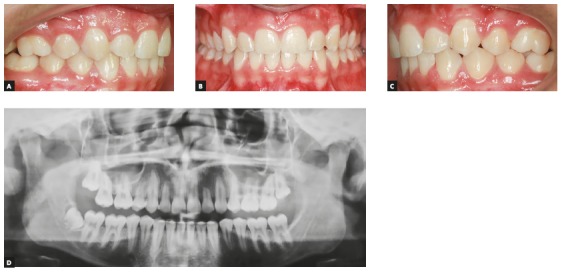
A, B, C) Intraoral posttreatment photographs. D) Posttreatment panoramic radiographic shows root length preservation of tooth #22.
